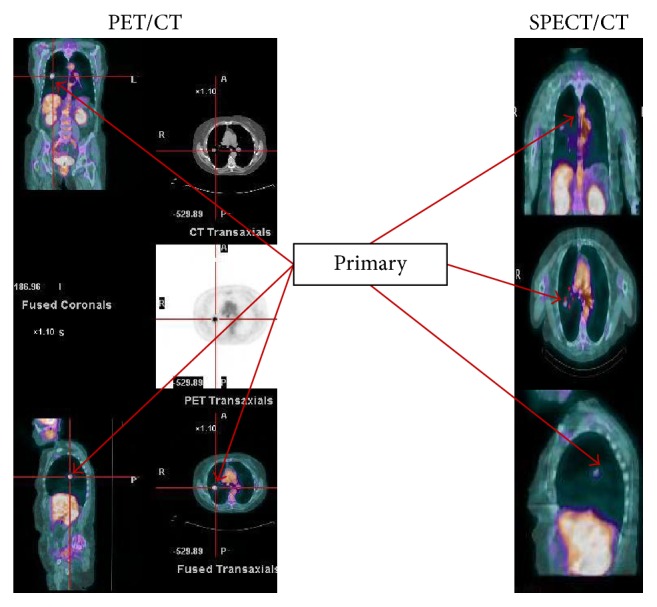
Comparative SPECT/CT: ^99m^Tc- EC-G and PET/CT: ^18^F- FDG image sets on a 68-year-old patient having a biopsy confirmed primary NSCLC.
